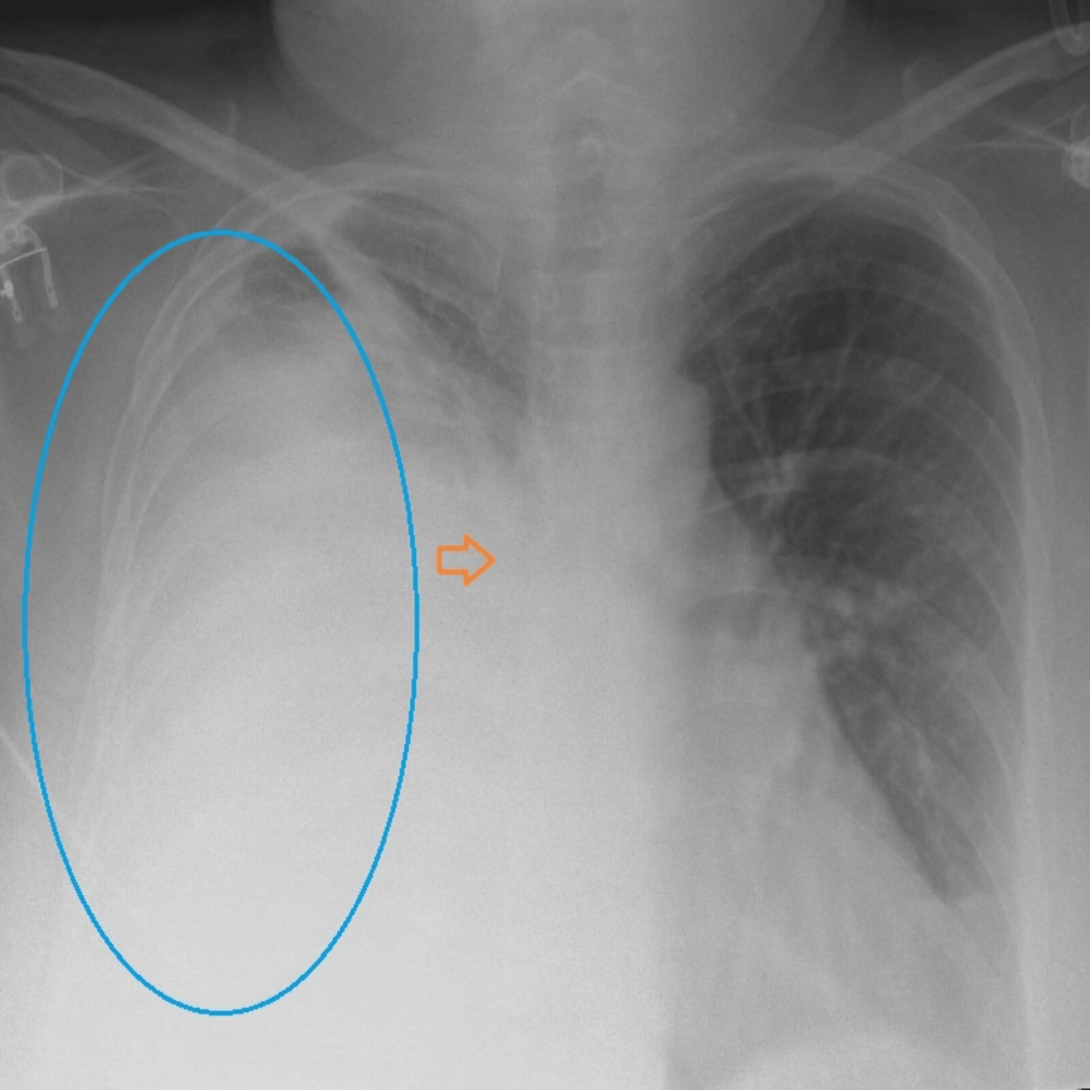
The chest X-ray shows a large right-sided white-out of the lung (shown in blue marking) with a loss of bronchial markings (orange arrow), indicating compression.
